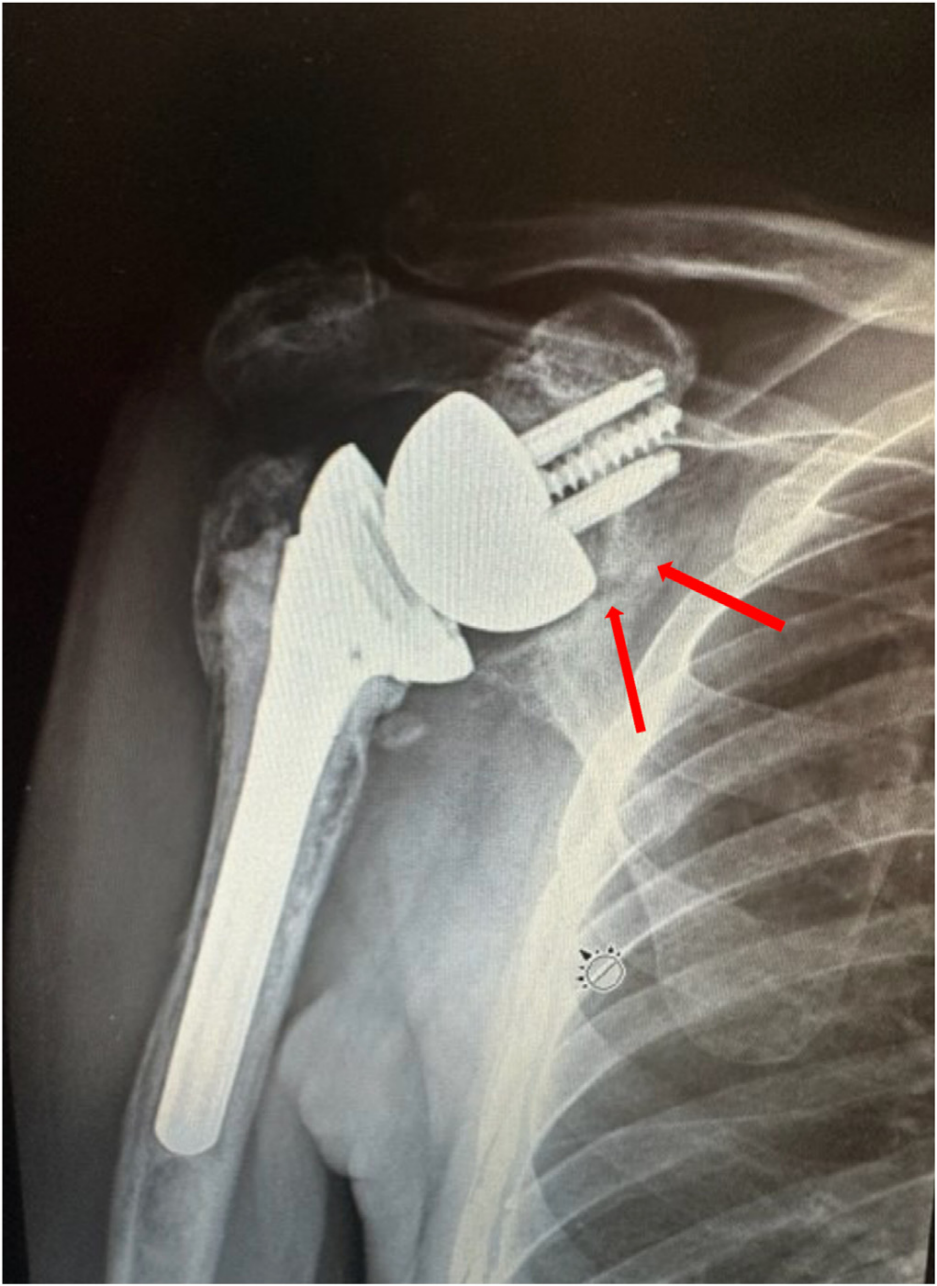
X-ray Grashey view demonstrating healing of the fracture at the scapular body.
